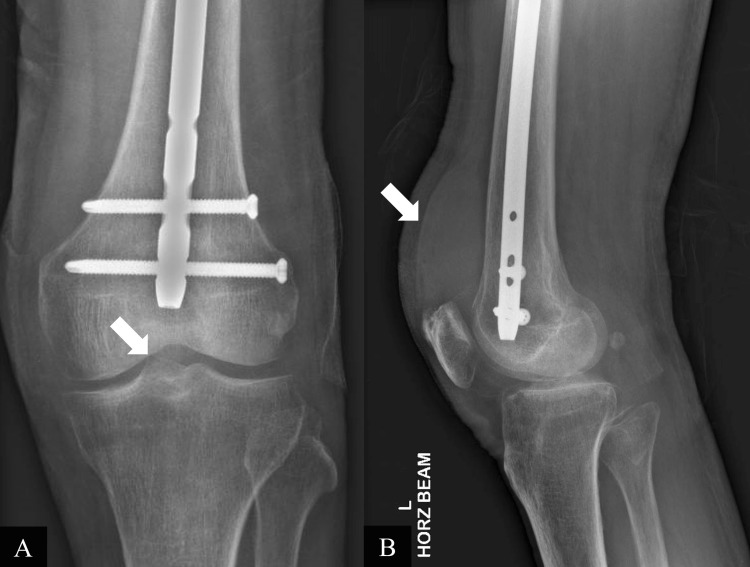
Anteroposterior (A) and lateral (B) radiographs of the left knee showing a hyperdense suprapatellar effusion with background patellofemoral and medial tibiofemoral compartment osteoarthritis.
